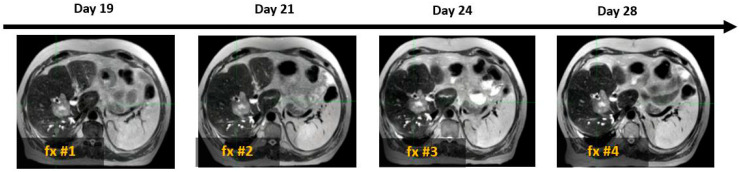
Example of four daily MR image sets used for online treatment plan adaptation targeting solitary colon cancer metastasis (white arrow) with SBRT (50 Gy in 4 fractions). Days 19, 21, 24, and 28 indicate the time elapsed since Ferumoxytol^®^ injection, corresponding to sequential SBRT fractionation (fx).
